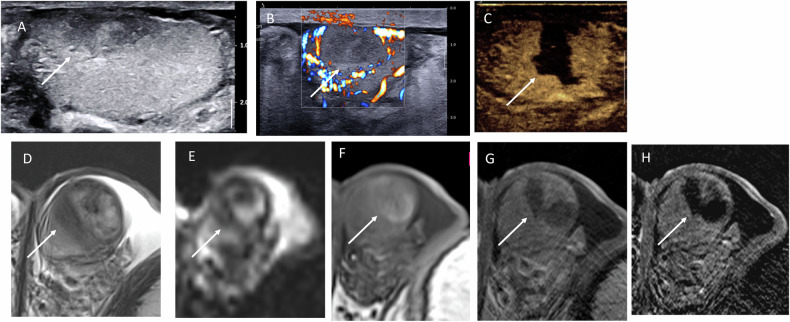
Segmental testicular infarction: US and MRI performed 48 hours after sudden-onset pain. A Hypoechoic lesion on B-mode US. B Peripheral hypervascularity on ultrasensitive Doppler US. C CEUS showing absence of contrast enhancement within a wedge-shaped lesion. D T2-weighted MRI showing a wedge-shaped hypointense lesion with well-defined borders. E ADC map showing diffusion restriction at the periphery. F T1-weighted MRI showing focal hyperintensity consistent with partial hemorrhagic transformation. G Post-contrast T1-weighted MRI showing absence of enhancement. H Subtraction image confirming lack of enhancement
